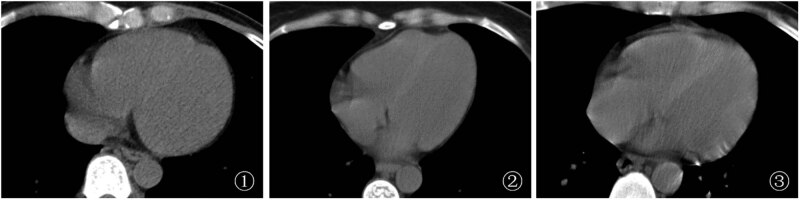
Chest CT images of a HIV-infected patient with anemia (1) A male patient, 44 years old, with mild anemia, HB = 109 g/L, LVD and RVD slightly lower than VSD; (2) A female patient, 42 years old, with moderate anemia, HB = 77 g/L, LVD and RVD lower than VSD; (3) A male patient, 46 years old, with severe anemia, HB = 40 g/L, LVD and RVD significantly lower than VSD.
